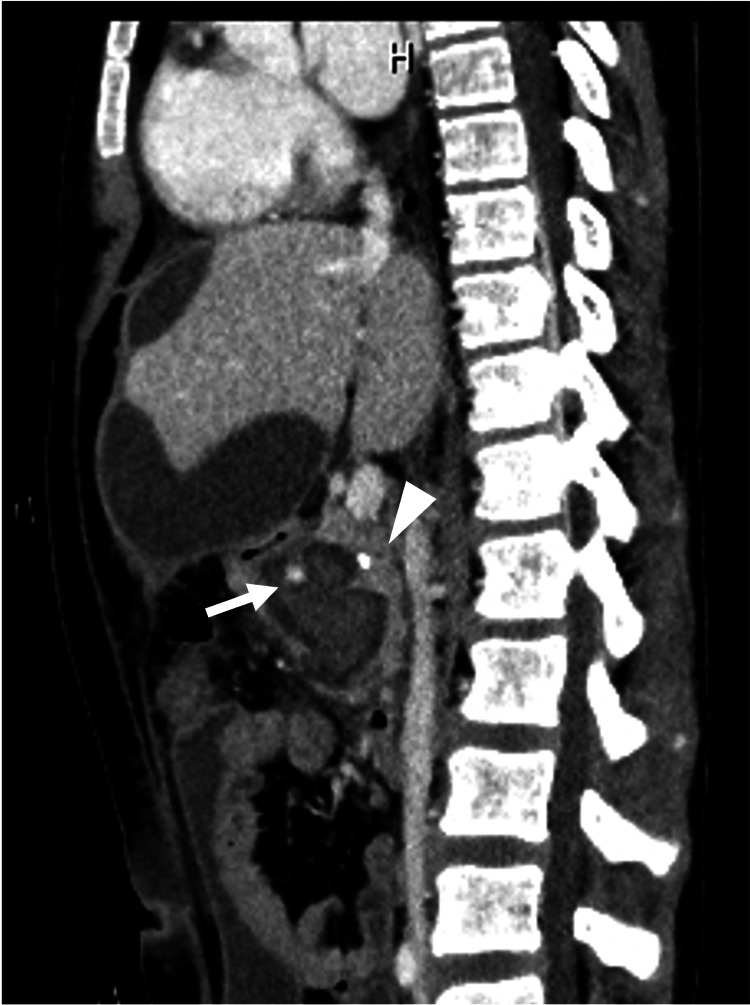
Contrast-enhanced computed tomography Pseudoaneurysm (white arrow) within the walled-off necrosis. The arrowhead shows a stent placed in the pancreatic duct.
